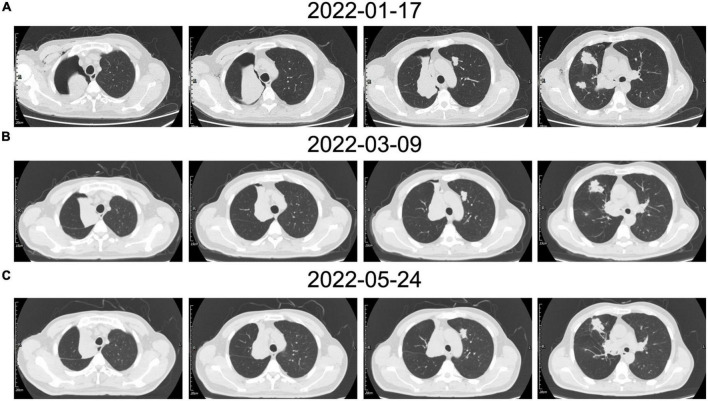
(A) The patient’s chest CT examination conducted for the first time showed multiple nodules located in the bilateral lobe, the right main bronchus was obstructed which caused pulmonary atelectasis in the right upper lobe. (B) The chest CT examination after vascular intervention showed recruitment of the superior lobe of the right lung. (C) In contrast to the previous chest CT, the most recent chest CT showed a reduction in tumor size.
